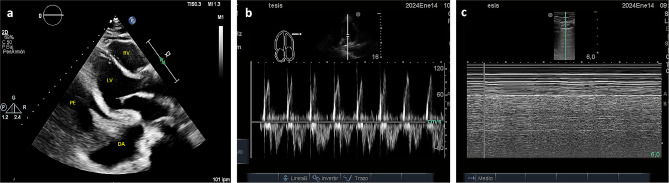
(a) Echocardiographic evaluation after drainage with a decrease in the effusion RV: right ventricle, LV: left ventricle, PE: pleural effusion, DA: descending aorta (b) Doppler through mitral valve showing no changes in peak E-wave during respiration. (c.) Absence of pneumothorax being evaluated by assessing pleural sliding and the presence of the seashore sign in the nearest pleural space that can be visualized
